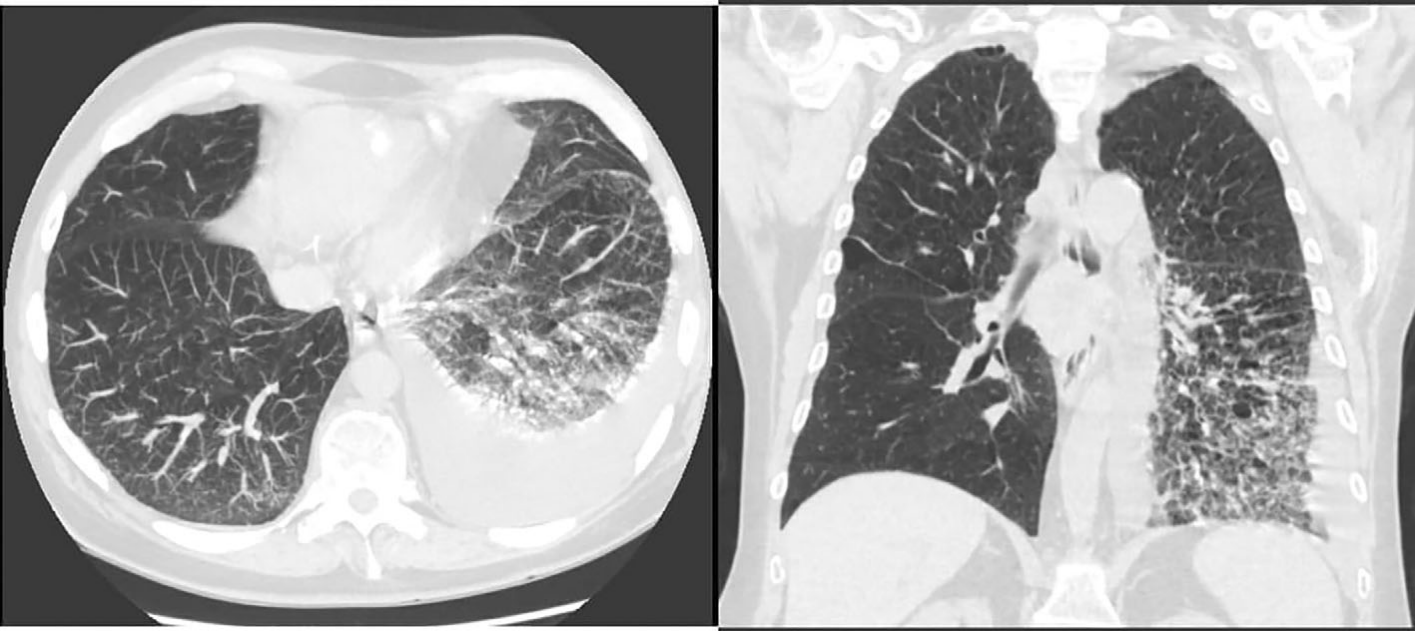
Transverse and coronal view of chest CT showing left‐sided pleural effusion with atelectasis
